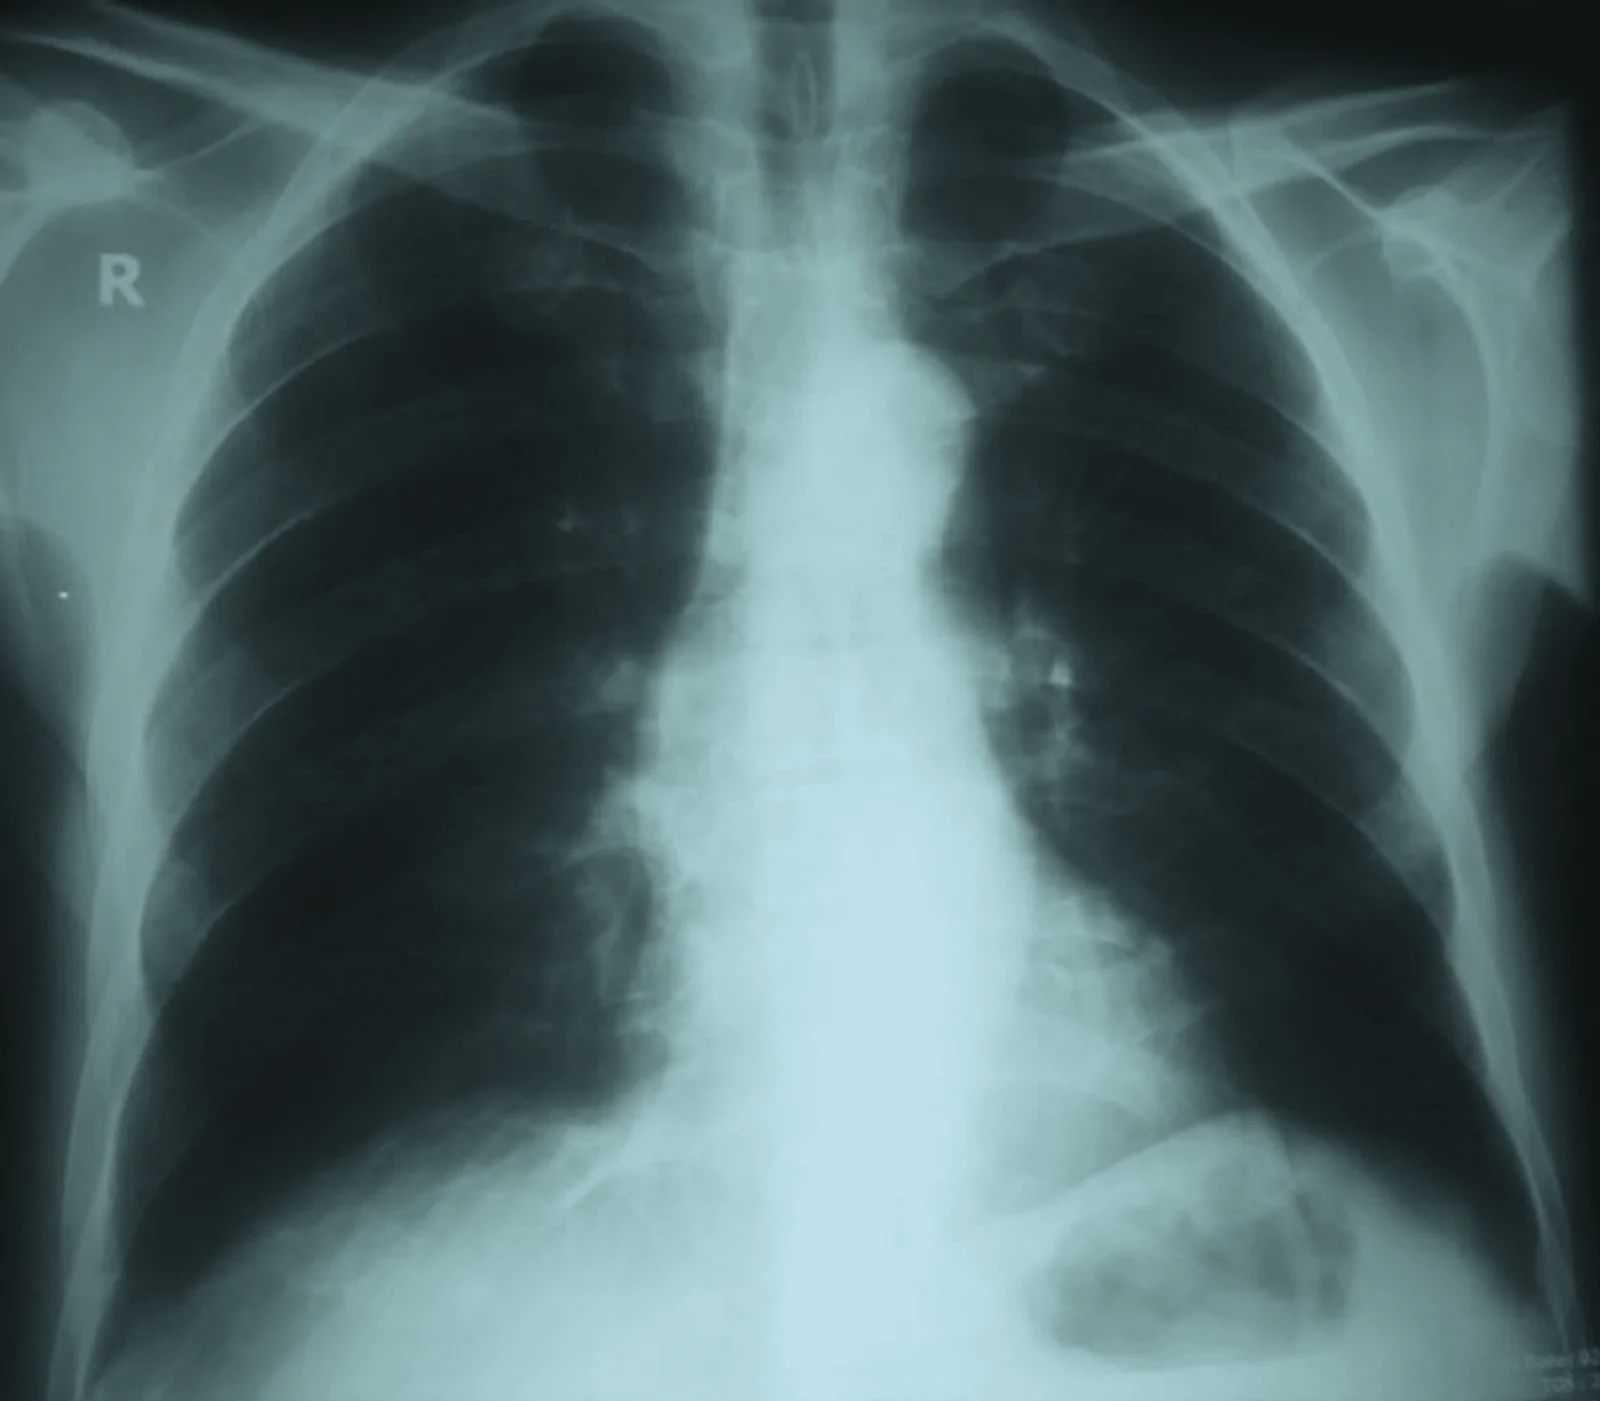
Normal chest radiograph. Chest radiograph demonstrating no significant cardiopulmonary abnormality. The cardiac silhouette is within normal limits, and no focal pulmonary infiltrate, pleural effusion, or other acute abnormality is identified.
